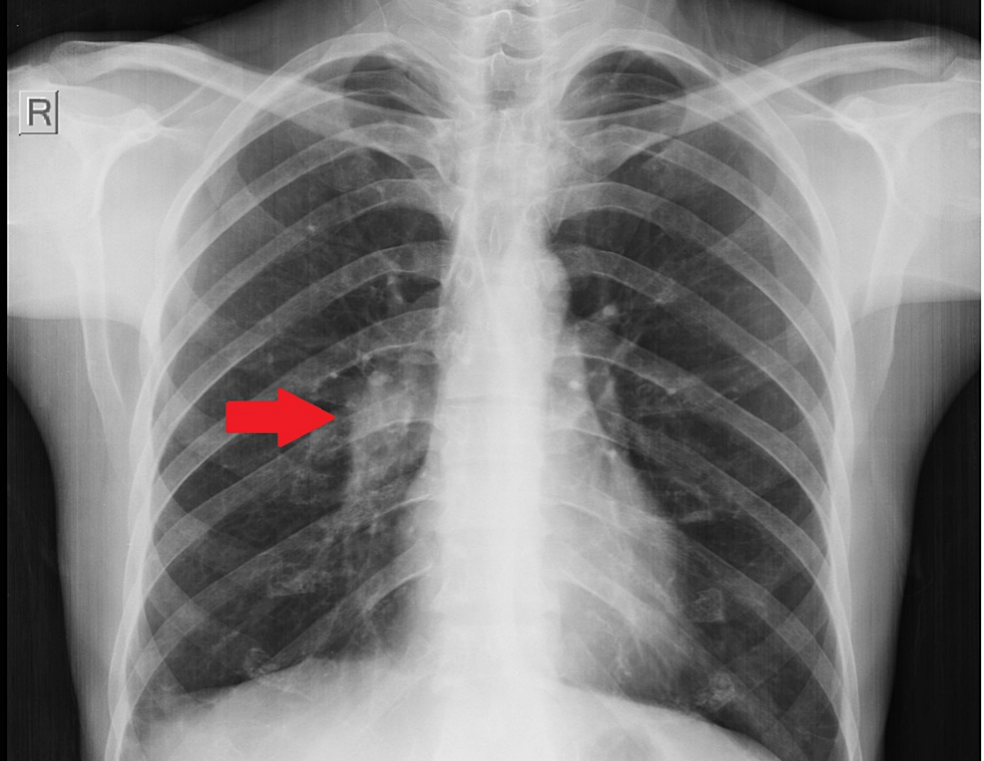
Chest X-ray on hospital admission The initial evaluation was done at the emergency ward with the chest X-ray revealing an enlarged right hilus, indicating a mediastinal or vascular pathology
